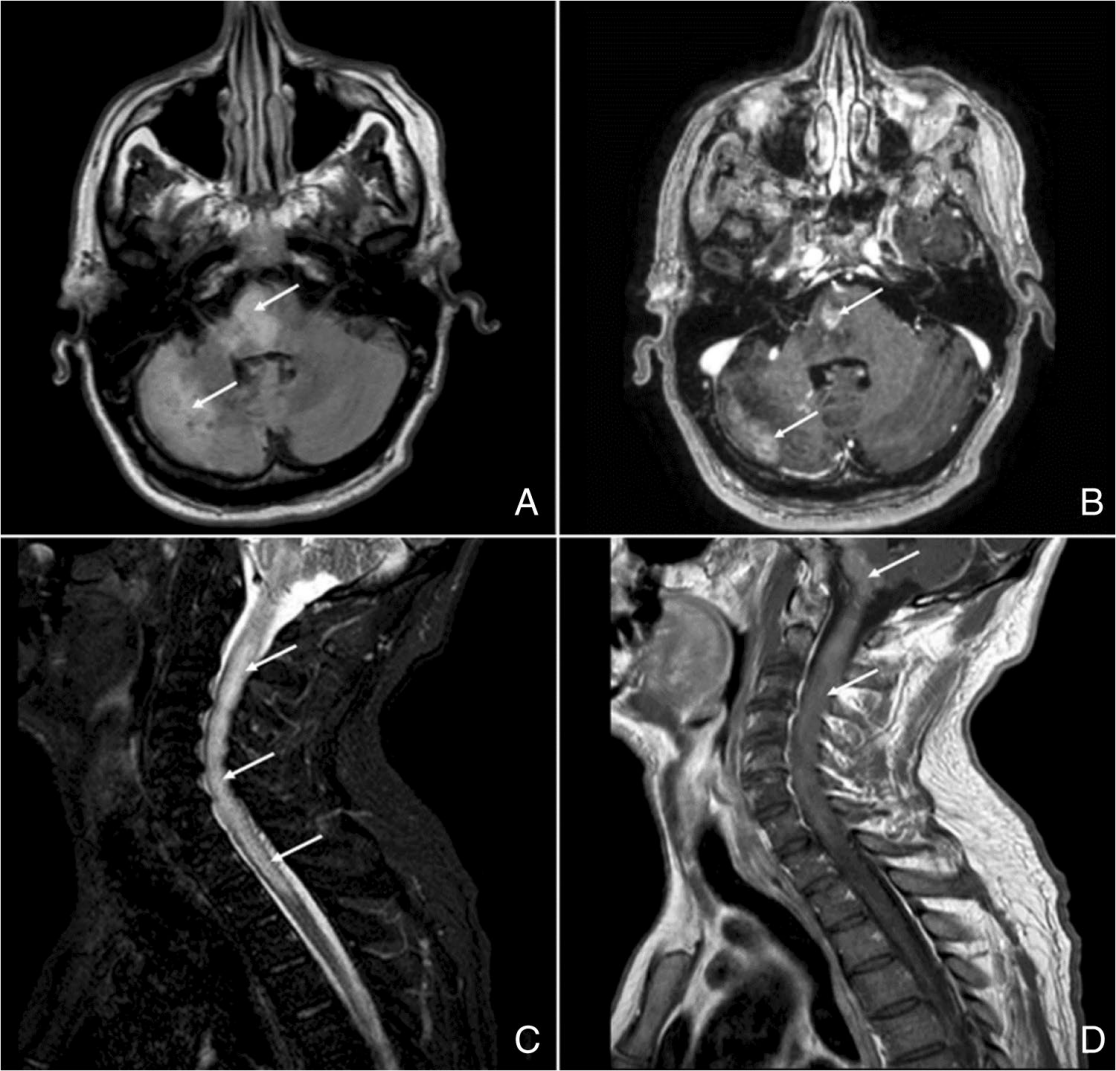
MRI at the diagnosis. A Axial FLAIR sequence shows hyperintensities in the pons and in the right cerebellar hemisphere (white arrows). B Axial T1-weight image obtained after gadolinium administration reveals enhancement of the same areas (white arrows). C Sagittal T2-weighted image shows intramedullary hyperintensity from the medulla oblongata to D2 vertebra level, with swollen cervical spinal cord (white arrows). D Gd-enhancement of the lesion shown in C (white arrows)
